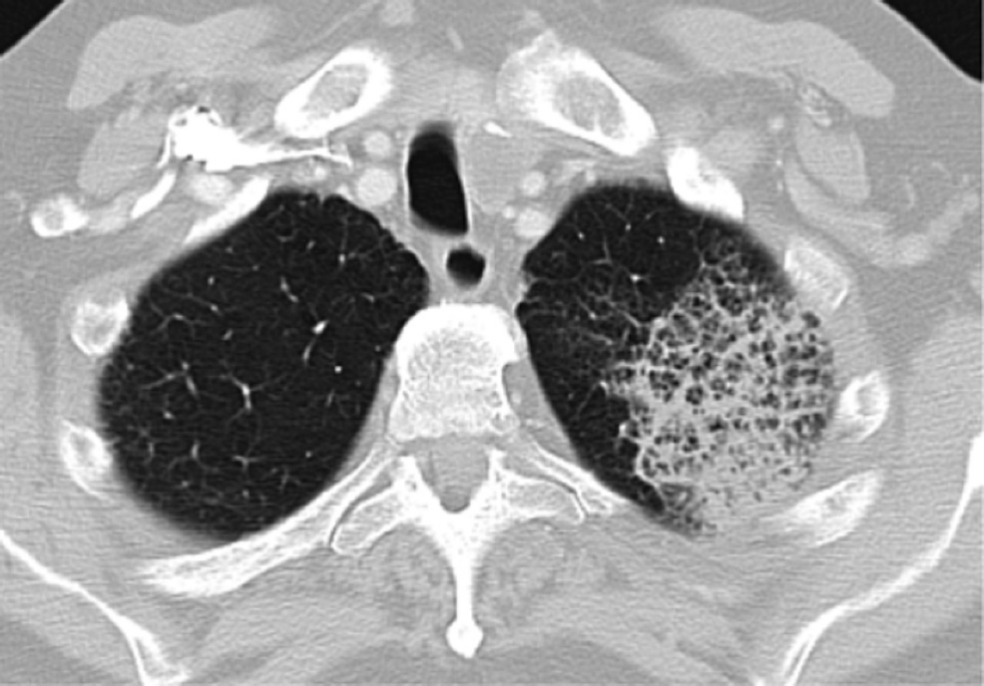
CT of chest revealing left upper lobe cavitary pneumonia.
